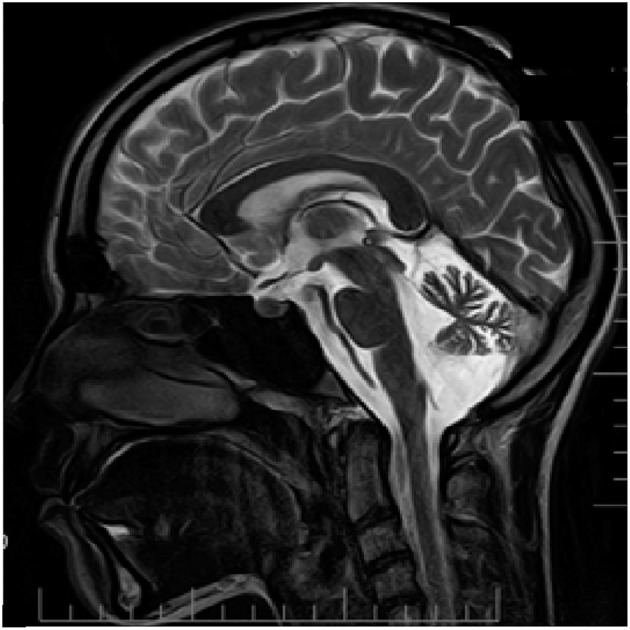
MRI examination (T2 weighted sagittal image) of patient D. Notice clear cerebellar atrophy. Patient information and technical text were closed.
